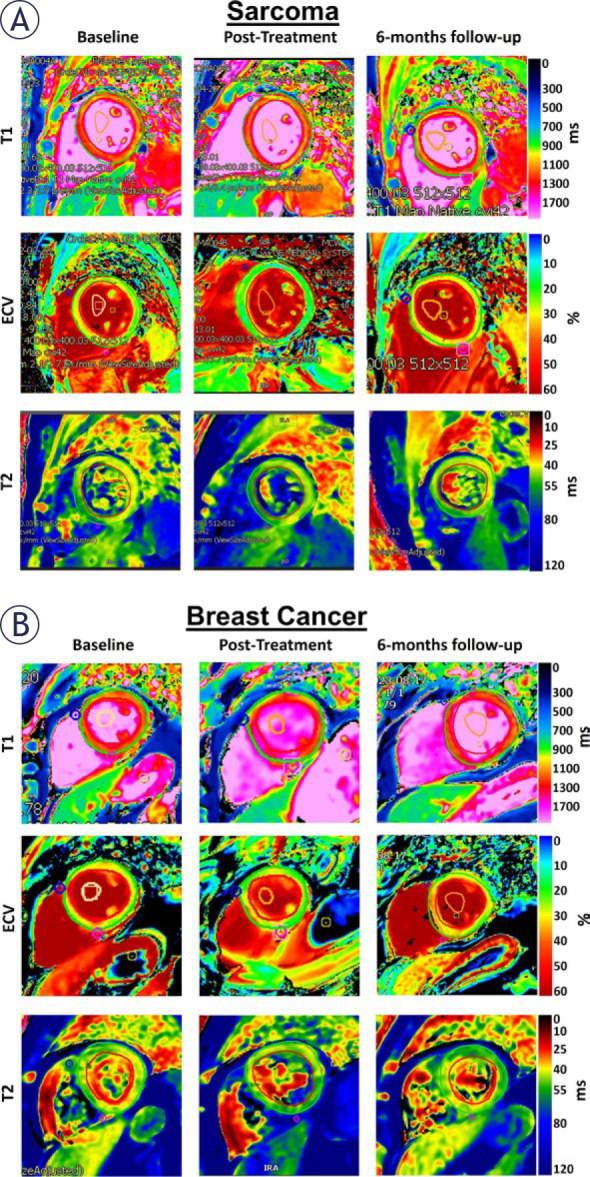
MRI tissue characterization maps in (A) sarcoma and (B) breast cancer. The figure shows myocardial T1, extracellular volume (ECV), and T2 maps. Note changes in the maps between sarcoma and breast cancer patients as well as between different study timepoints (baseline, posttreatment, 6-months follow-up).
